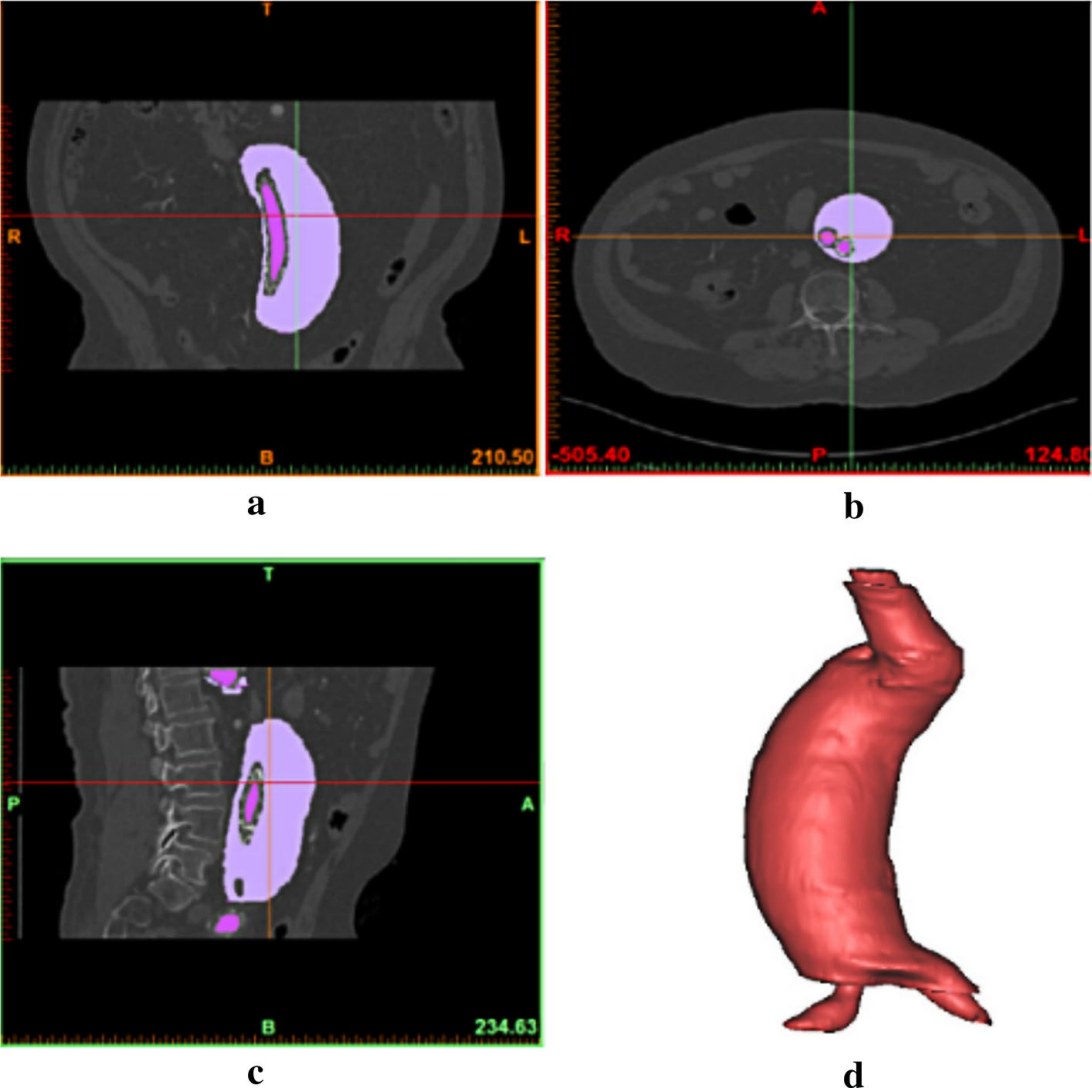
CT images and the reconstructed model with Mimics. a coronal view of CT images, b axial view of CT images, c sagittal view of CT images, d reconstructed model with Mimics
